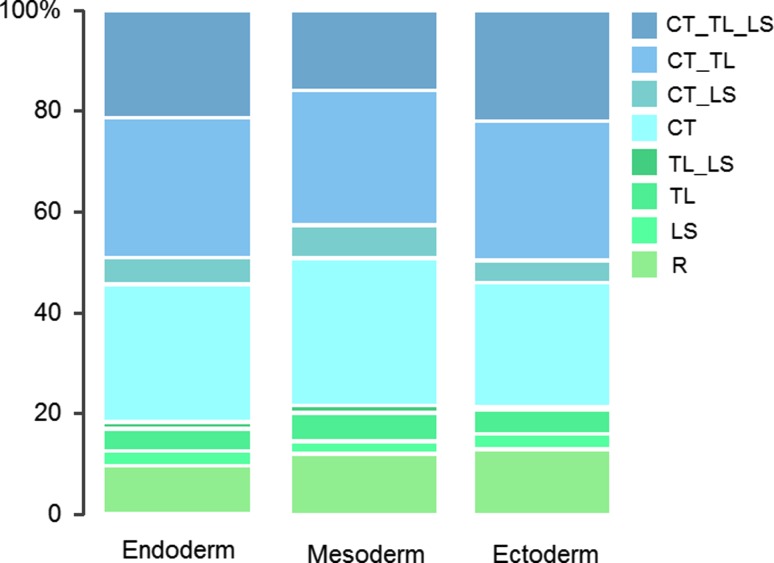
Frequencies of different vertebral patterns for fetuses with malformations of a particular germ-layer, i.e. endoderm, mesoderm, ectoderm. Vertebral patterns are indicated with R, LS, TL, TL_LS, CT, CT_LS, CT_TL, CT_TL_LS, see Fig. 2 for an explanation. There is no significant effect of the germ-layer of origin on the frequencies of vertebral patterns
